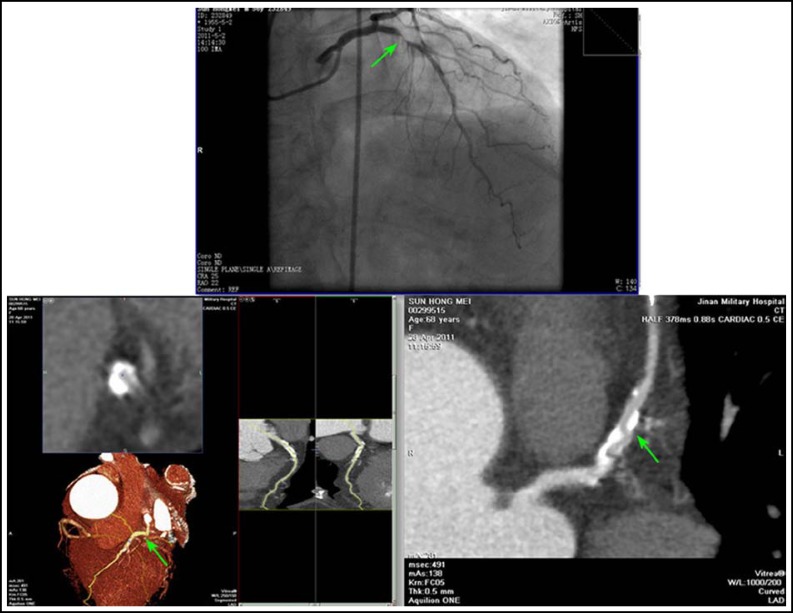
Severe calcification effected judgment of coronary stenosis degree by CTA. CAG showed severe stenosis of 99 percent in the proximal of LAD, CTA showed the stenosis of about 30%.
